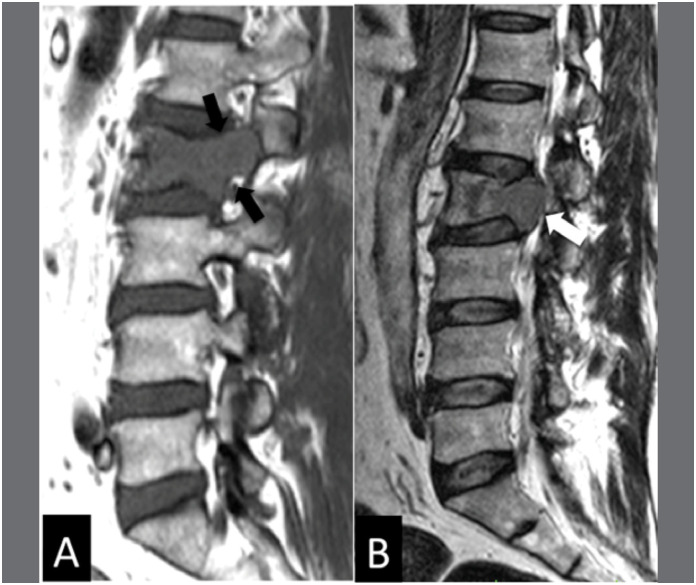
MRI sagittal sequences T1 (A) and T2 (B) exemplifying a case of malignant fracture of the vertebral body of L2. Notice the involvement of the pedicle and the hyposignal throughout the vertebral body (black arrows) and the bulging of the posterior wall (white arrow).
